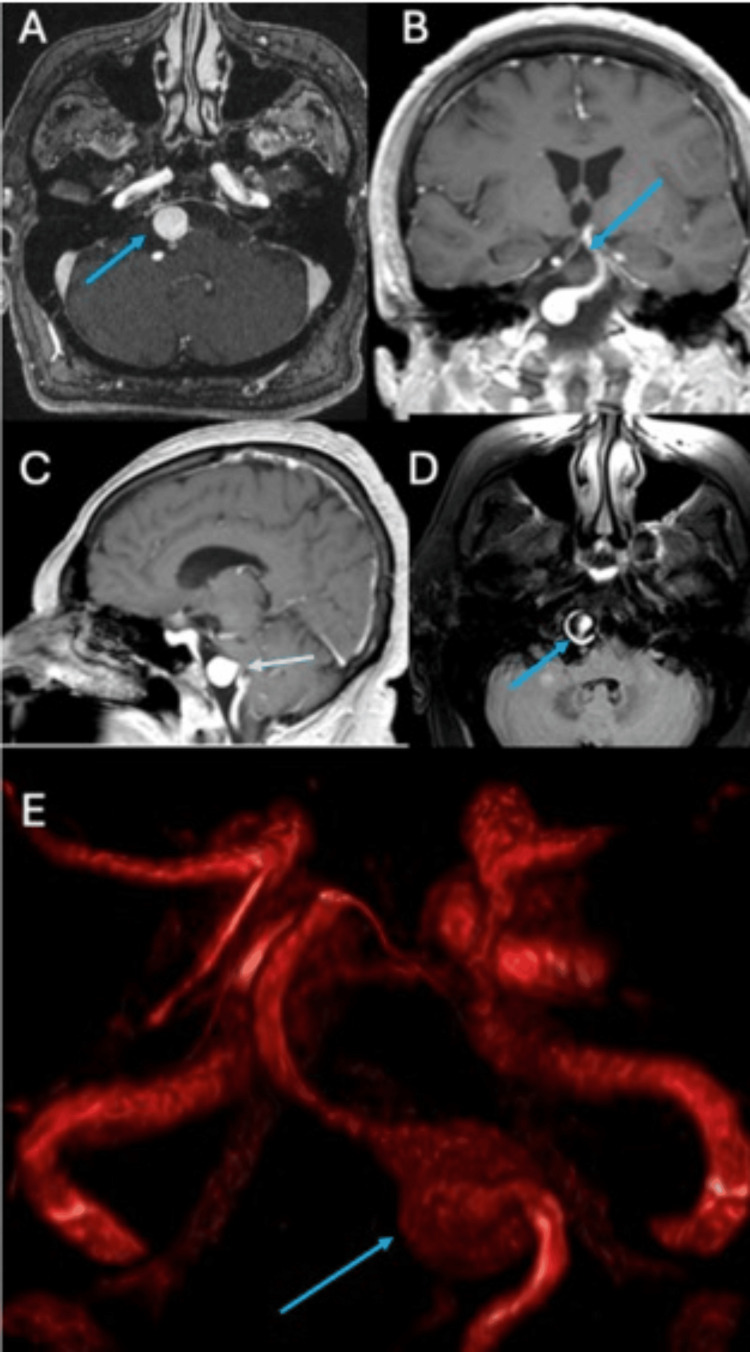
Magnetic resonance images with T1 gadolinium-weighted sequences in axial (A), coronal (B), and sagittal (C) views, along with fluid-attenuated inversion recovery-weighted axial section (D), showing enhancement at the vertebrobasilar level corresponding to an aneurysm. Three-dimensional reconstruction showing the anterior and posterior cerebral circulation, with the presence of a right vertebrobasilar aneurysm and an absence of flow in the left vertebral artery (E).
